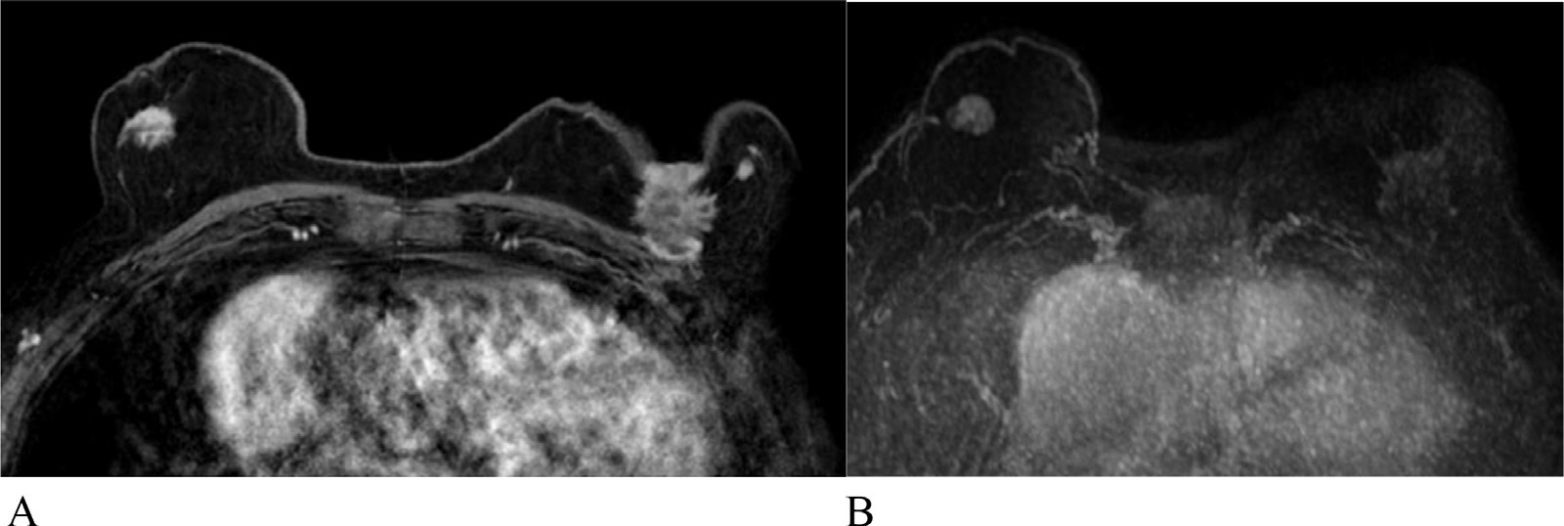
Full-protocol dynamic contrast enhanced (DCE) breast MRI with ultrafast sequence. (A) Subtraction images demonstrate an oval enhancing mass with irregular margins in the right breast, consistent with invasive ductal carcinoma with micropapillary features and an irregular mass with spiculated margins in the left breast with nipple and chest wall invasion, consistent with invasive breast carcinoma of no special type and (B) Both lesions show rapid contrast uptake on ultrafast sequence; however, the enhancement is more avid for the right breast malignancy.
